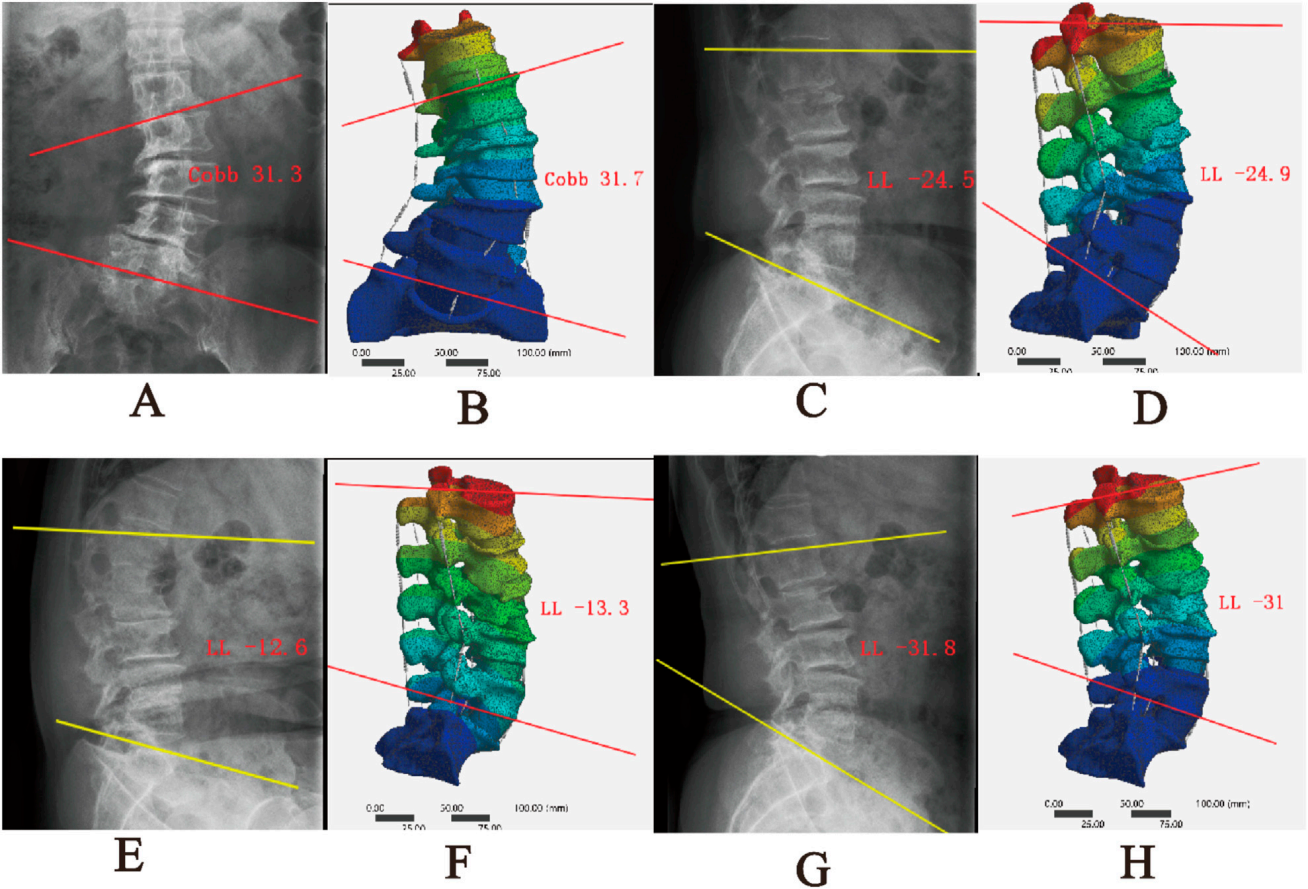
Comparison between the DLS model and actual radiographs; (A,B) Anteroposterior view; (C,D) Lateral view; (E,F) Flexion; (G,H) Extension.
